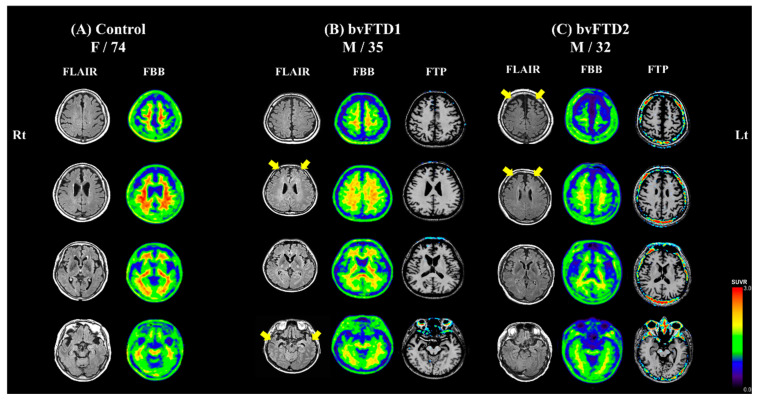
Brain images of participants. Axial views from top (in the first row) to bottom (in the last row) section of the brain are shown. (A) The 74-year-old normal control subject showed no atrophy on fluid-attenuated inversion recovery (FLAIR) image. Florbetaben (FBB) PET showed no abnormal uptake, indicating no significant amyloid-beta deposition. (B) The 35-year-old sporadic behavioral variant frontotemporal dementia (bvFTD) patient showed a marked atrophy in the fronto-temporal area on FLAIR image (yellow arrows). His FBB PET showed no abnormal uptake. Flortaucipir (FTP) PET showed no abnormal uptake, indicating no significant paired helical filament tau deposition. (C) The 32-year-old sporadic bvFTD patient showed a prominent atrophy in the frontal area on FLAIR image (yellow arrows). FBB PET and FTP PET showed no abnormal uptake.
